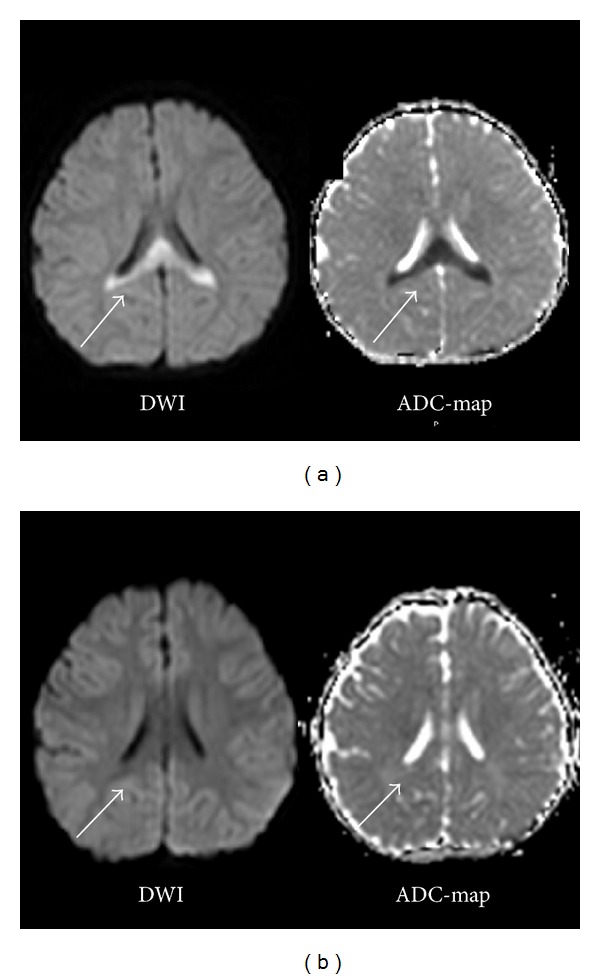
(a) Head MRI on day of admission (day 4 of illness). MRI was performed on the day the seizures and disturbance of consciousness appeared. DWI reveals a hyperintense signal in the splenium of the corpus callosum and a hypointense signal on the ADC-map. (b) Head MRI performed 8 days after admission (day 12 of the illness). Head MRI (DWI, ADC-map) shows that the abnormal signal detected in the splenium of the corpus callosum had disappeared.
